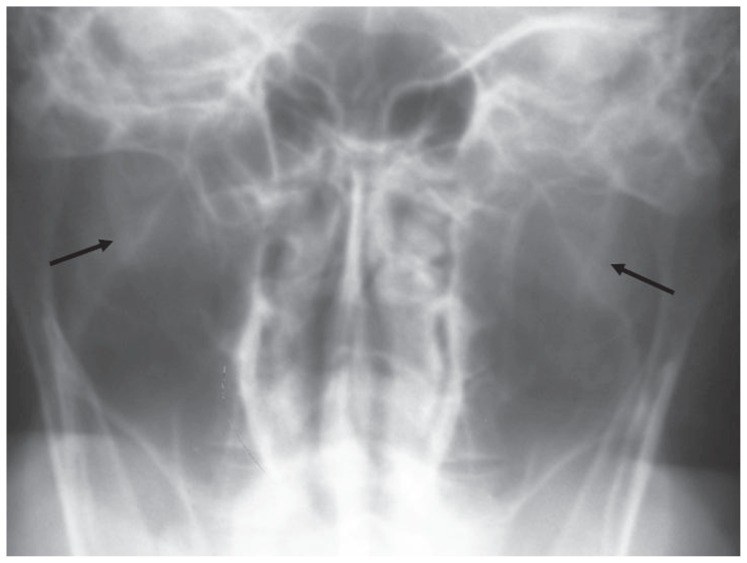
Towne radiograph shows bilateral elongated styloid processes (right: 29 mm, left: 30 mm).
